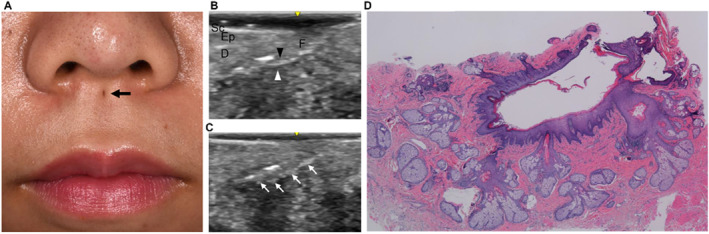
Ultrasound is a minimally invasive examination method. Previous examinations have shown that the most common standard methods used to reveal the distal aspect of the congenital labial sinus, fistula probe or methylene blue dye, are highly invasive and ultrasound is less invasive. Of course, there are cases where CT is necessary, but CT carries the risk of radiation exposure.
